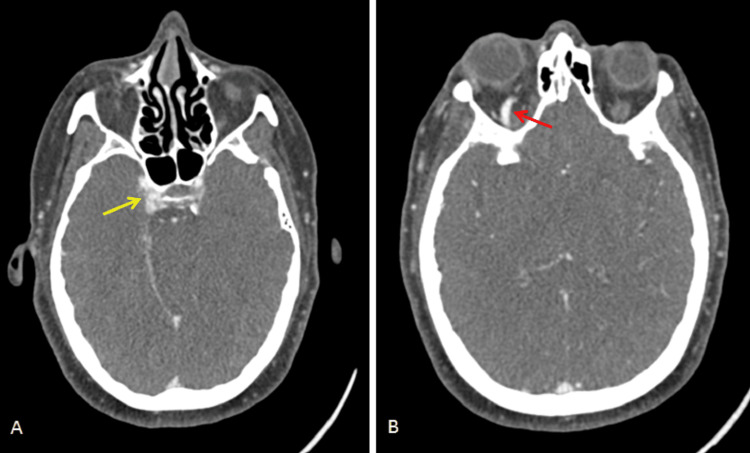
Head angio CT images of the patient. (A) axial view showing the enlargement of the right cavernous sinus due to its arterialization (yellow arrow) and (B) axial view showing the engorgement of the right superior ophthalmic vein due to its arterialization (red arrow).
